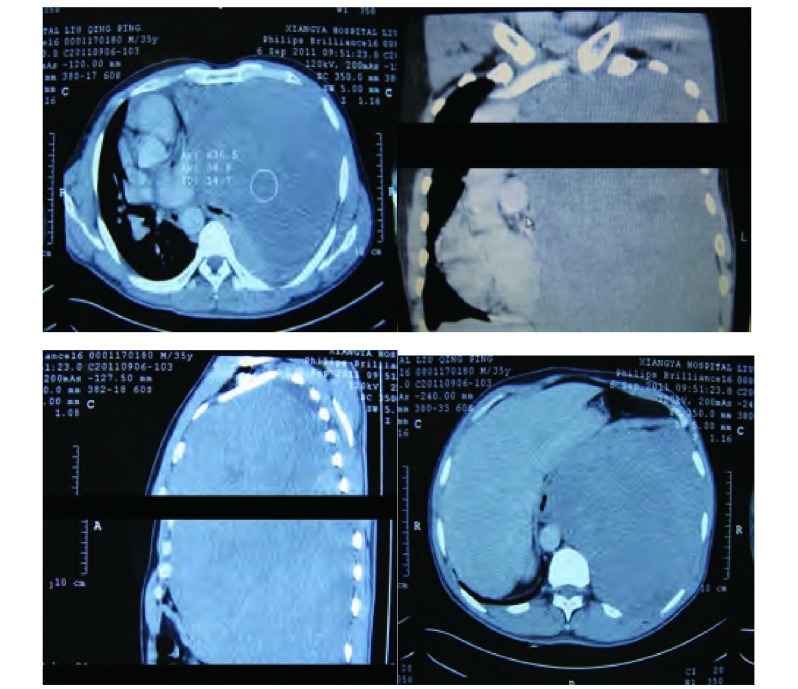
术前CT示肿块填满左侧胸腔，下极达肝脏平面，左肺被完全压缩。 Preoperative CT shows that the mass fills the left thoracic cavity with the low pole in the plane of the liver and the left lung is compressed completely.
